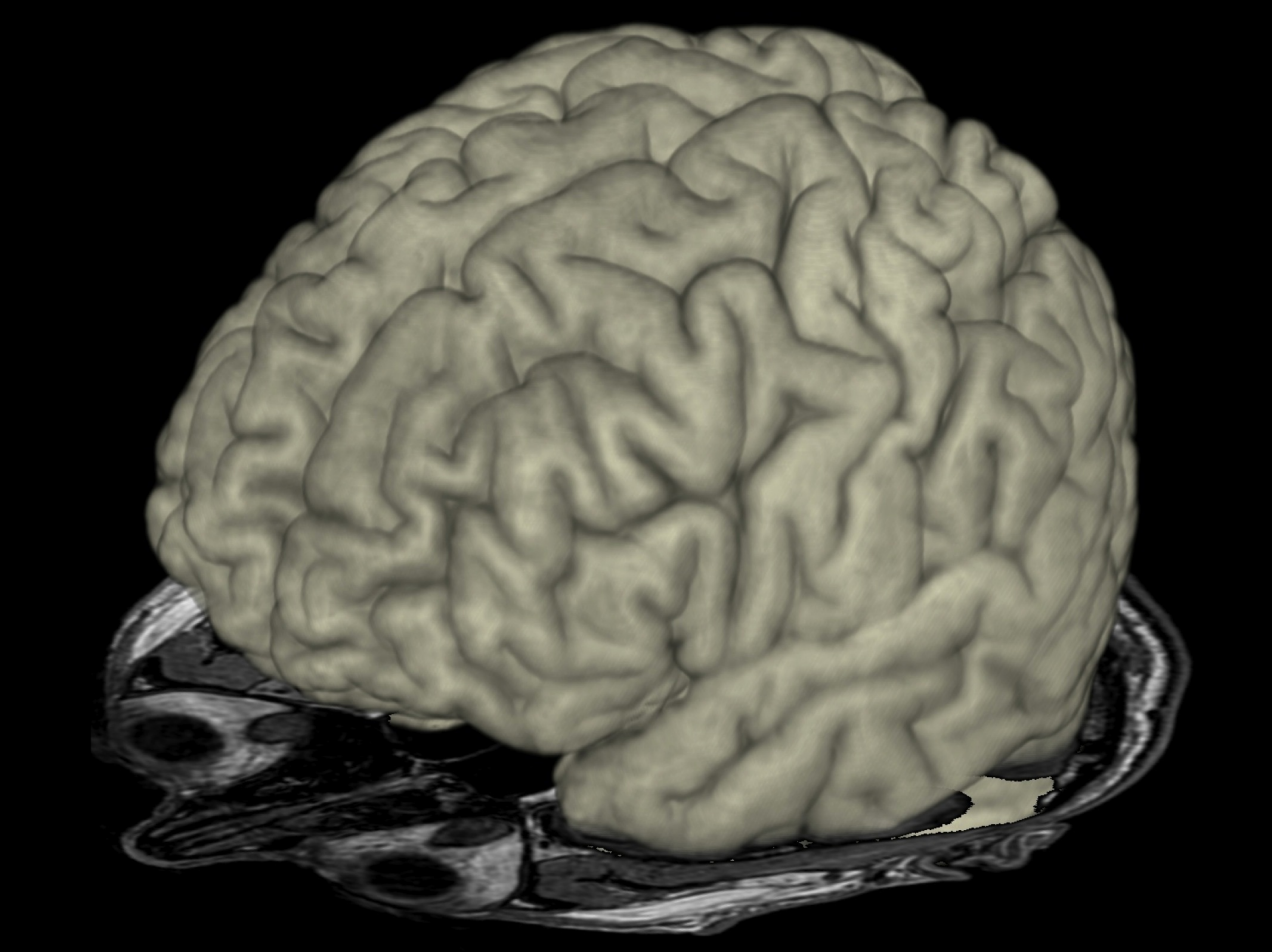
Three-dimensional magnetic resonance imaging (MRI) Three-dimensional MRI of the patient's brain showing no abnormalities
